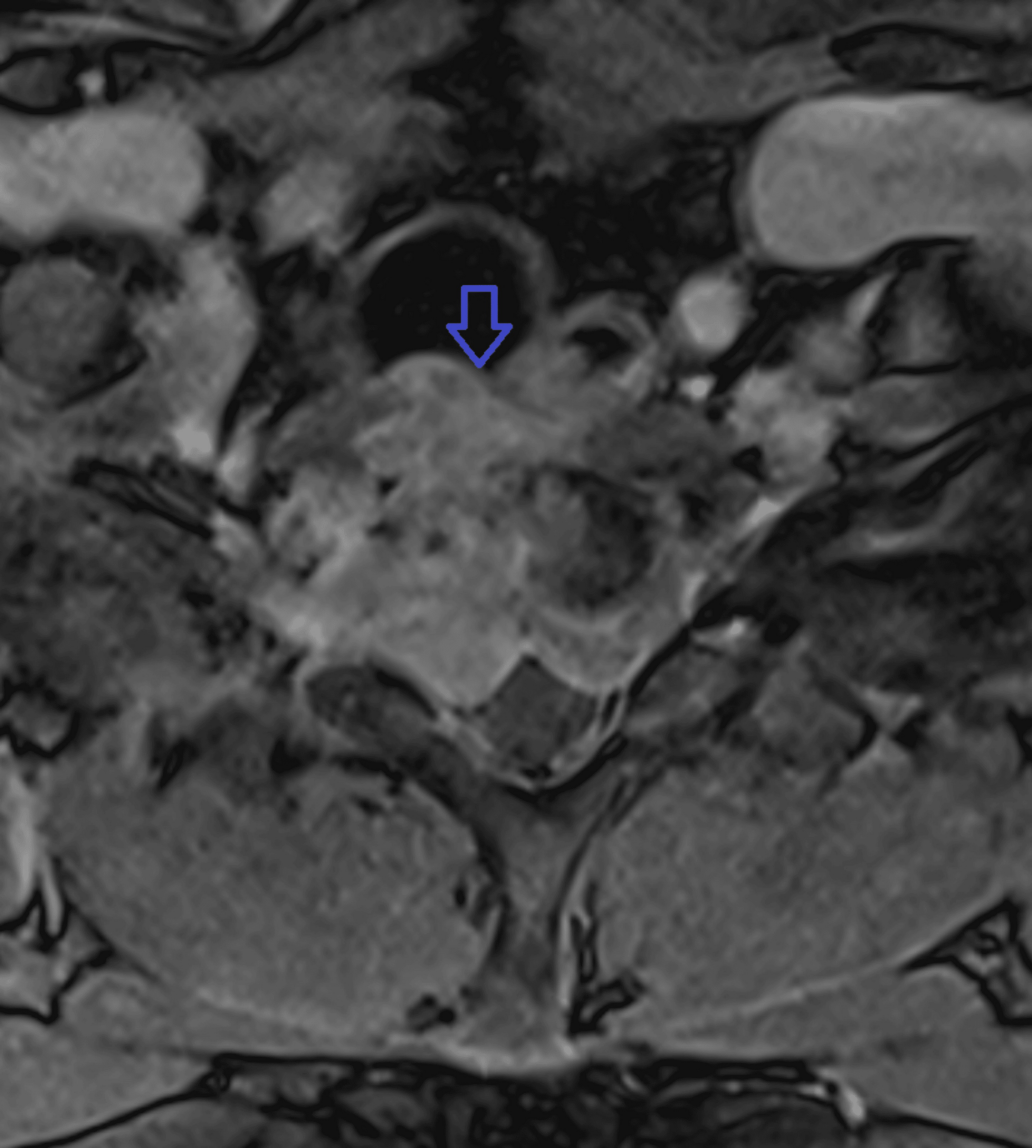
Repeat axial T1 post-contrast MRI demonstrating progression of the disease with near-complete destruction of the C7 vertebra due to disseminated coccidioidomycosis
